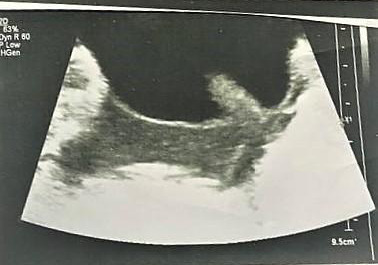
An in-utero ultrasonography at 30 weeks of gestation showing multiple cystic
